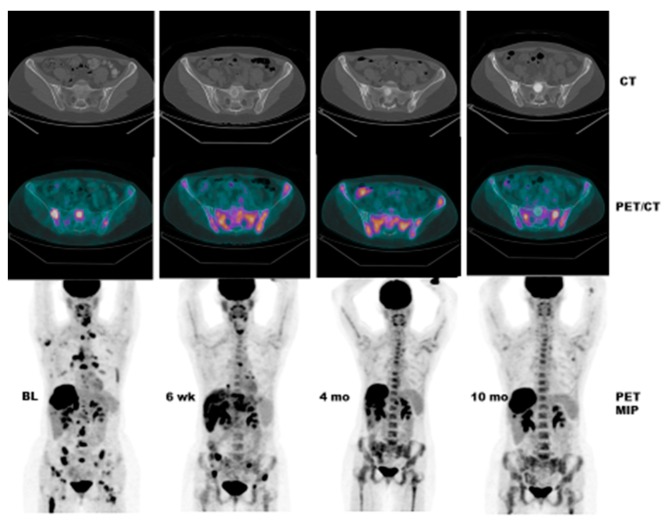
Pre- and Post FDG PET in a 47 y/o female with metastatic breast Cancer and multiple osseous metastases. Baseline study shows focal sites of activity in the bone marrow space, without discernible anatomic abnormality. About 6 weeks after starting therapy, there is diffuse marrow activation; however, there is relative loss of normal marrow activity where tumor was previously seen, and there is new sclerotic change at those sites. About 4 months after starting therapy, there is still diffuse marrow activation with loss of normal marrow activity where tumor was, and increased sclerosis on CT images. Unfortunately, about 10 months after starting therapy, she relapsed, with new focal sites of activity, without anatomic abnormality (similar to baseline study), whereas previously tumor sites have become densely sclerotic and remain without activity to suggest active tumor.
